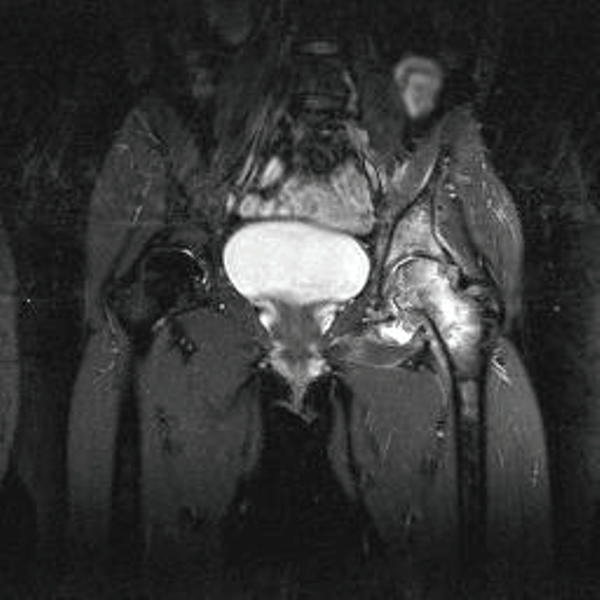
MRI scan made 2 years earlier already shows oedema of the bone marrow, joint effusion and some erosive changes of the left hip joint.(STIR-image; TR 5700.0 ms, TE 30.0 ms)
